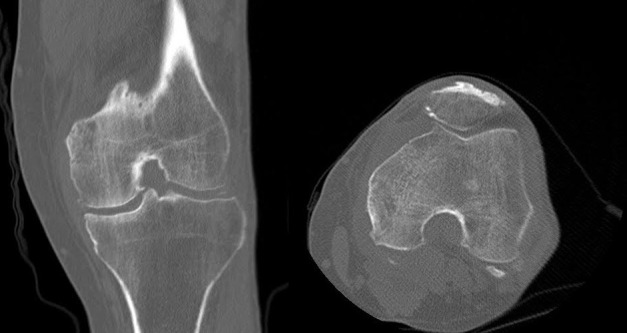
Coronal and axial CT images in bone window
